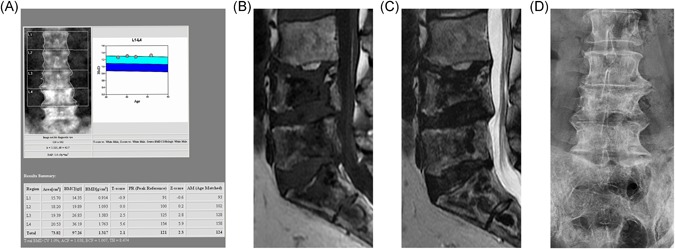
The lumbar spine of a patient with GD and recurrent bone crises despite therapy. All images taken several years after last acute bone crisis. (*A*) DXA image. Note the increased BMD in L3 and L4. These vertebrae were not excluded from the report, despite the variation in BMD among vertebrae, contrary to best practice. The total lumbar vertebral BMD reported is therefore artifactually elevated. (*B*) T1‐weighted MR image showing widespread, low‐signal geographic changes in L4, L5, and the sacrum, features indicative of osteonecrosis. Central endplate depression is also seen. (*C*) T2‐weighted image showing double‐line sign indicative of osteonecrosis best seen in S1 and S2 segments and areas of low signal in the bodies of L4 and L4. Low signal in both T1‐ and T2‐weighted images indicates osteosclerosis. (*D*) Plain anteroposterior lumbar spine radiograph in the same patient show osteosclerosis of the body of L4. The increased density at L4 and L5 is therefore a result of osteosclerosis, consequent upon osteonecrosis, although a contribution from endplate depression cannot be excluded
